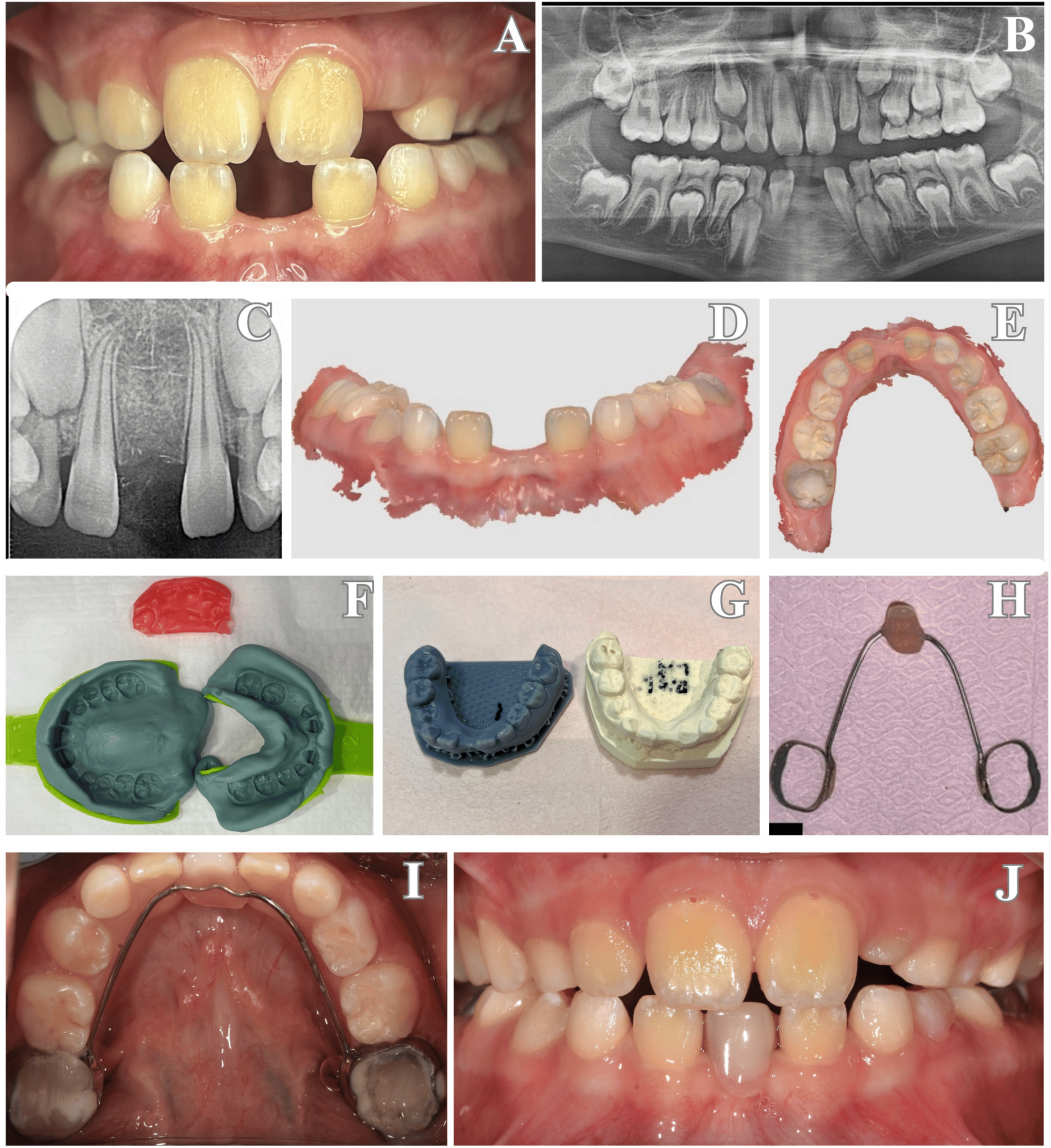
(A) Intraoral view showing the congenital absence of the mandibular permanent central incisors. (B) Panoramic radiograph confirming bilateral agenesis of teeth #31 and #41. (C) Periapical radiograph for further evaluation of the anterior mandibular region. (D, E) Digital impressions and 3D scan images for appliance planning. (F) Conventional impression using type A silicone and wax bite registration. (G) Working model obtained from the impressions. (H) Fabricated single-tooth pontic integrated into a mandibular lingual arch appliance. (I, J) Post-insertion intraoral views of the final appliance in occlusion and from the occlusal perspective.
